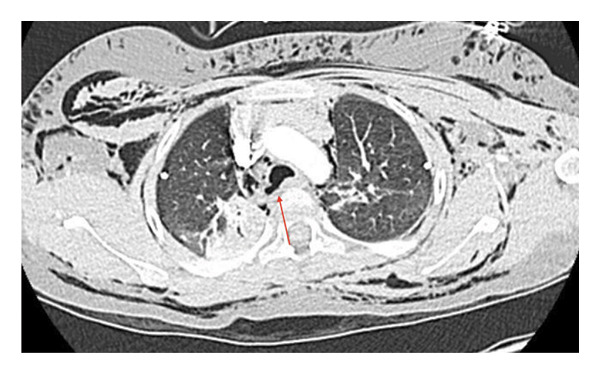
Initial chest computed tomography (CT) performed at our center displaying tracheal defect (arrow).
